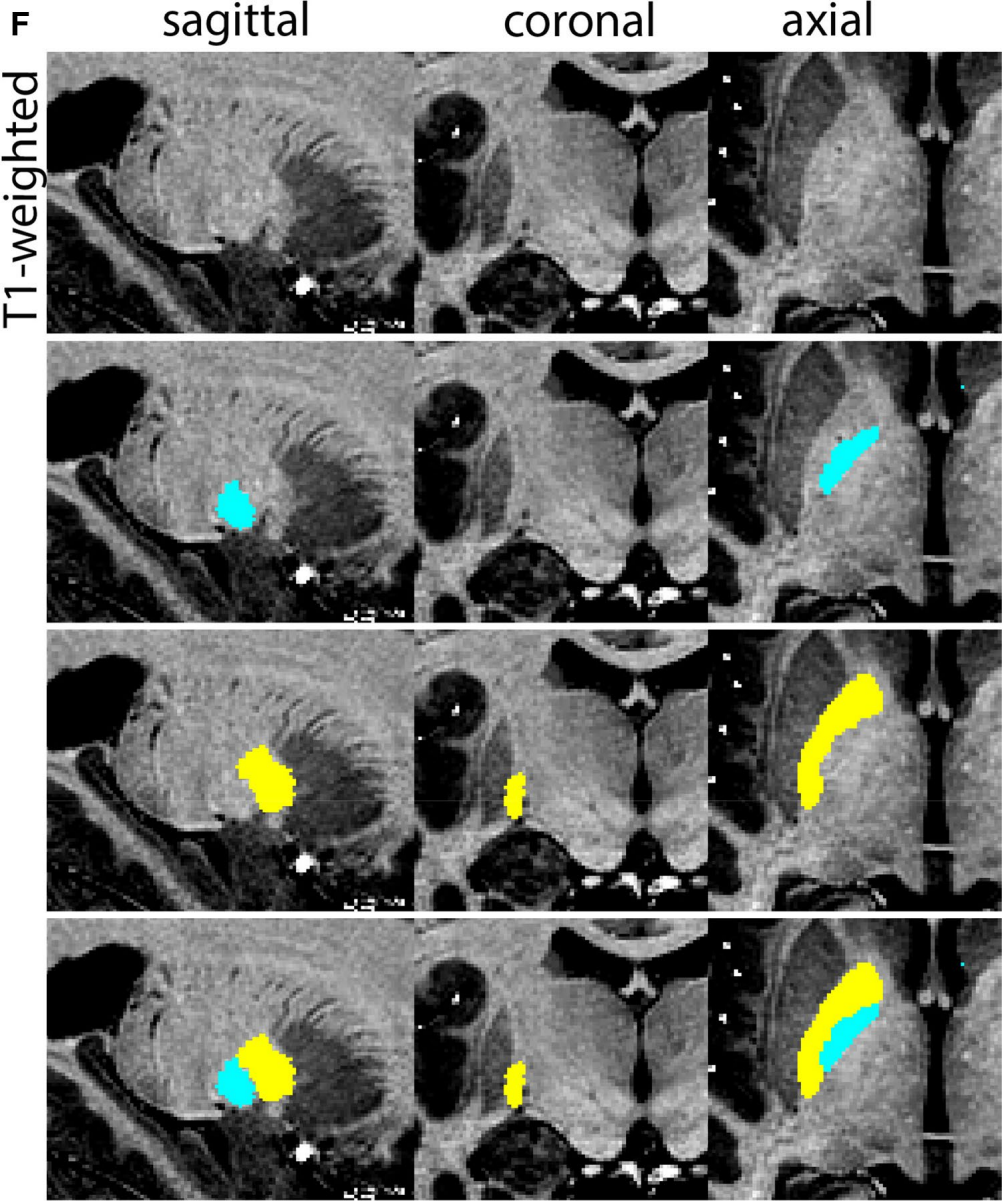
a Globus pallidus internal and external segment (GPi and GPe) in a central view on QSM. Top row QSM contrast. Note the separation between the GPi and GPe by the hypointense mml. Yellow = GPe, Light blue = GPi. b Globus pallidus internal and external segment (GPi and GPe) at the level of the mamillary bodies on a T1-weighted contrast. Top row T1-weigthed contrast. Note the boundary between the GPe and the hypointense striatum which is located lateral to the GPe, and the lack of contrast between the GPi and in in the T1-w contrast. Yellow = GPe, Light blue = GPi. c Globus pallidus internal and external segment (GPi and GPe) in the coronal plane on the QSM contrast at the level where the anterior commissure crosses the 3V (not visible on QSM). Top row QSM contrast. Note the absence of the GPi from the coronal (middle) panel at these rostral levels. Note the increased visibility of the dorsal GPi/e border as a result of the hypointense appearance of the internal capsule. Yellow = GPe, Light blue = GPi. d Globus pallidus internal and external segment (GPi and GPe) in a rostral view on T1-weighted contrast at a level in which the anterior commissure crosses the 3V. Top row T1-weigthed contrast. Note the limited contrast between the GPe, the internal capsule and the anterior commissure at this level. Note the limited visibility of the ventral pallidum. Yellow = GPe, Light blue = GPi. e Globus pallidus internal and external segment (GPi and GPe) in a caudal coronal view on a QSM contrast. Note the clear visibility of other iron-rich structures including the STN and SN at this level. Top row QSM contrast. Note the limited contrast between the GPe, the internal capsule and the anterior commissure at this level. Yellow = GPe, Light blue = GPi. f Globus pallidus internal and external segment (GPi and GPe) in a more caudal view on a T1-weighted contrast. Note the reduced visibility of other iron-rich structures including the STN and SN at this level as compared to the QSM contrast in Fig. 4a. Top row T1-weighted image, note the clear visibility of the border between the Str and the GPe. Yellow = GPe, Light blue = GPi
